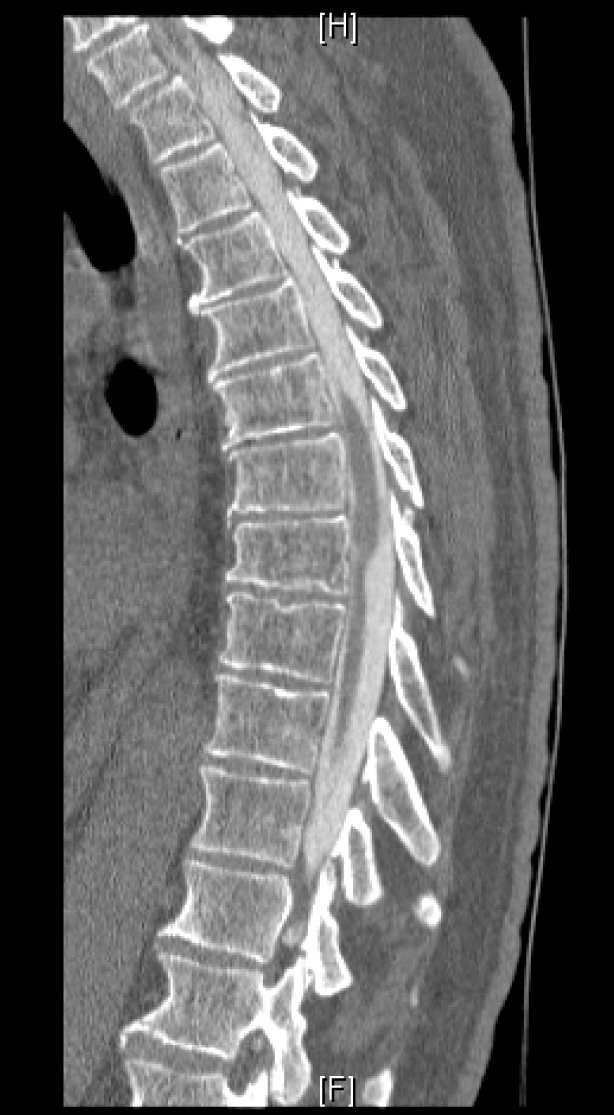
Computed tomography with contrast (a 67-year-old male patient).
